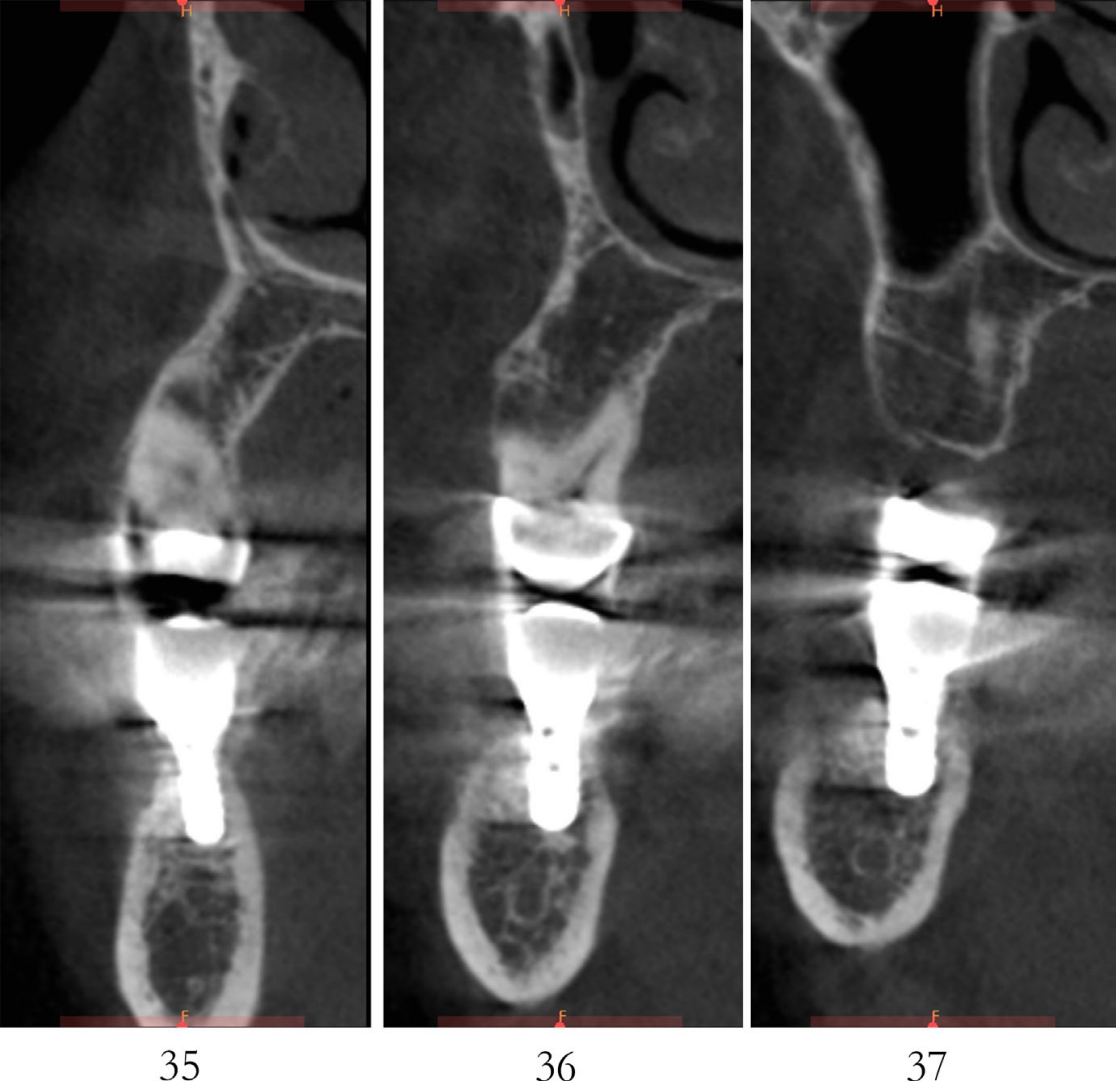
CBCT scanning the day at final restoration
